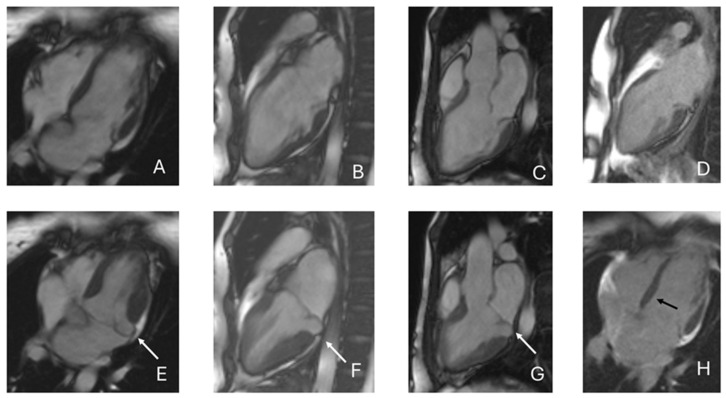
Cardiac magnetic resonance imaging. Cine in 4 (A), 2 (B), and 3 chambers (C) in diastole (upper row) and systole (bottom row). The aortic root has a diameter of 41 mm at the Valsalva sinus. The right ventricle with an ejection fraction of 43%. In (D–H) inversion- recovery sequence. In (E–G) the white arrow shows a submitral aneurysm, In (E) with subtle mid wall late and in (H) enhancement in the septum (black arrow).
